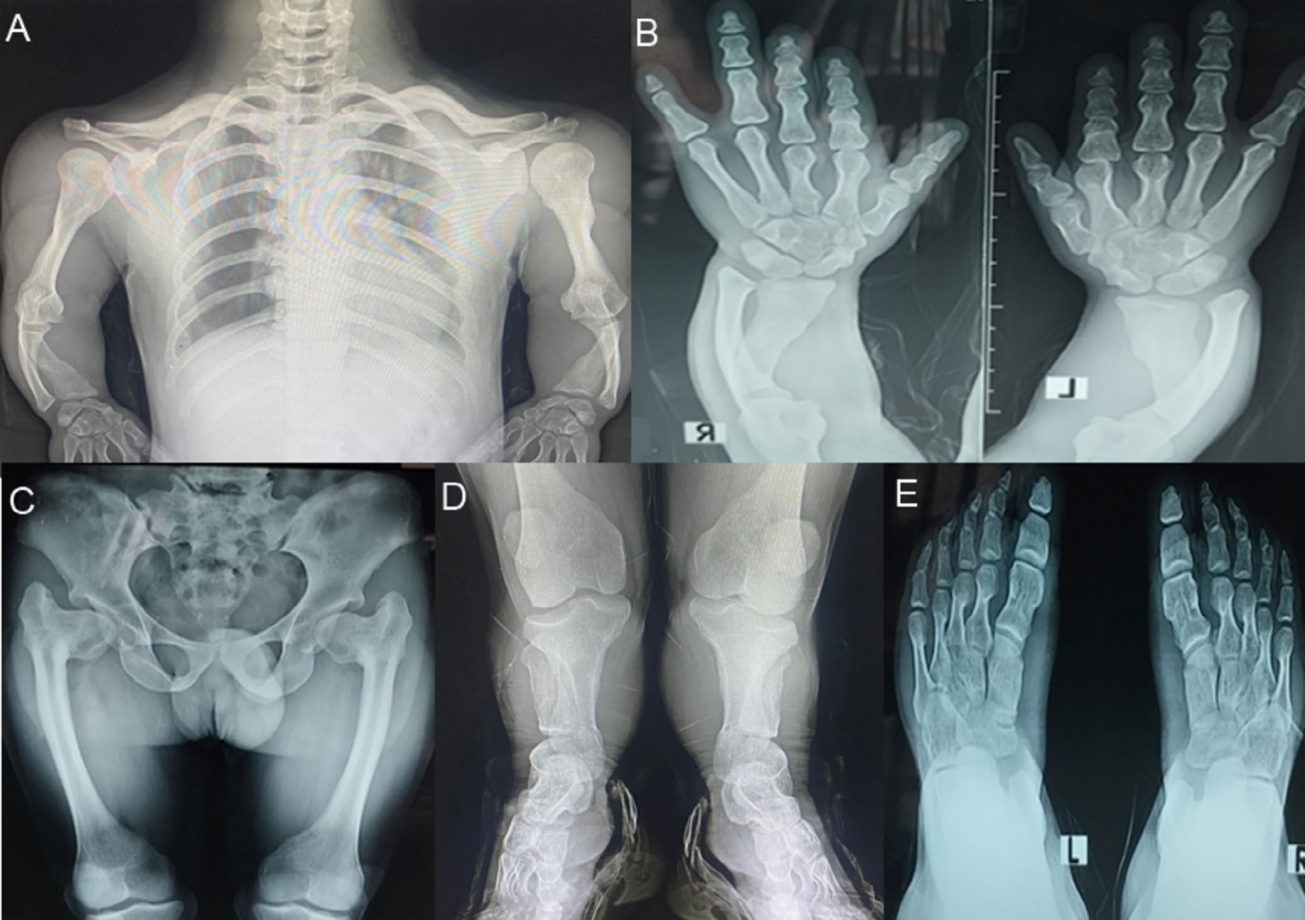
(A) Chest radiographs of IV‐1 showing narrow thorax, short ribs and clavicles. Short humerus bone with cone shaped epiphysis at the proximal end. Elbow joints depicting dislocated radial head and increased inclination angle of humerus head. Bilateral Varus angulation at the radial and ulnar bones with medlung like deformity at the distal radioulnar joint. (B) Hands radiographs indicating hypoplasia of middle phalange of little finger, fusion of middle and distal phalanges of 1st and 2nd finger and thumb, shorter metacarpal bones, and subluxation of ulnocarpal joint. (C) Pelvic region showing dysplastic acetabulum coxa vara with greater traochanter over growth, lateral pelvis tilted to right side, egg‐shaped capito femoral epiphyses with a short femoral neck, thin femoral shaft with narrow medullary cavity, hyperplastic distal femur, and genu vara (D) Extremely short tibia and fibulae with irregular proximal tibial metaphysis and cone shaped distal tibial epiphysis (E) short metatarsals with conical tear drop epiphysis, accessory navicle foot deformity, and fused middle and distal phalanx of fifth toe bilaterally.
